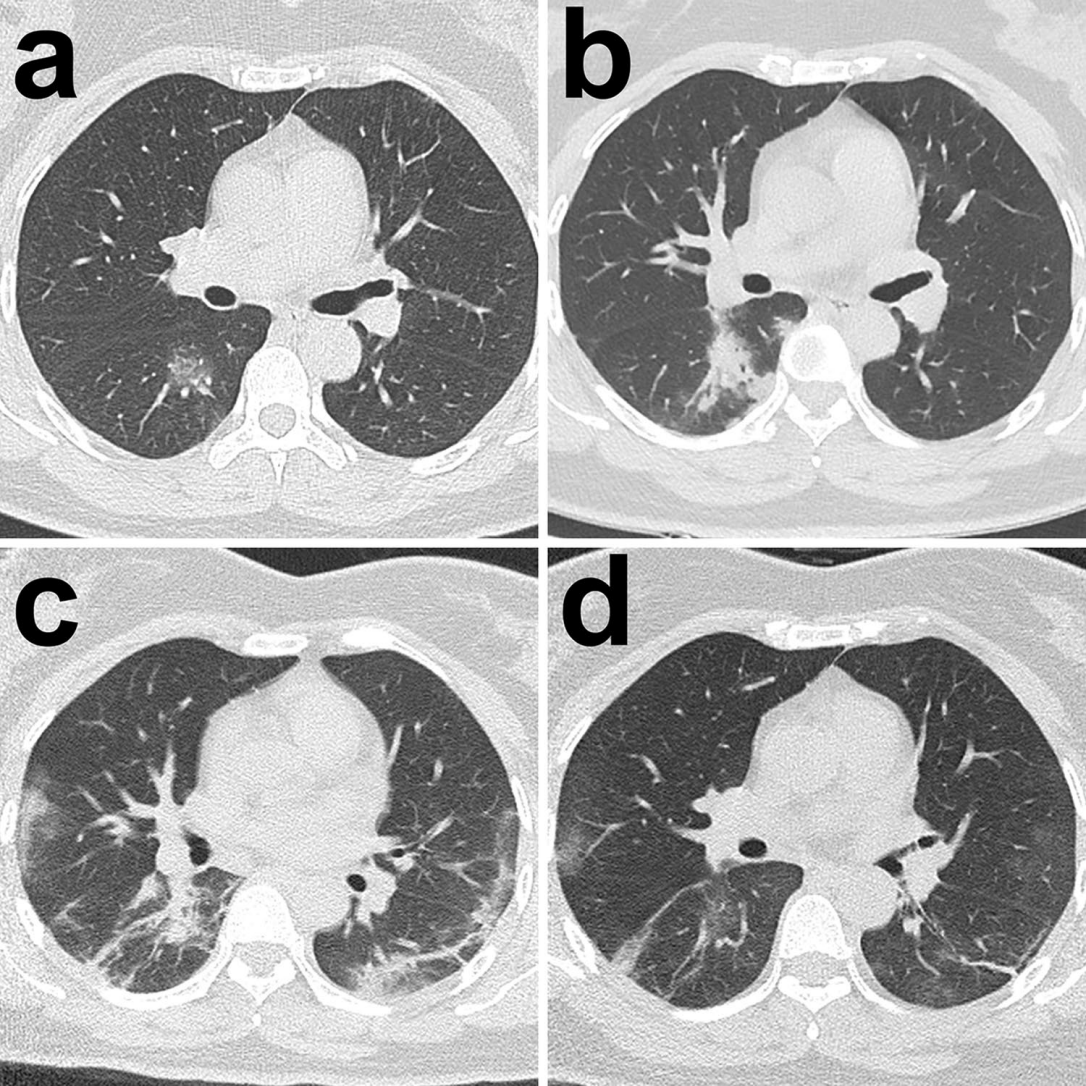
Typical radiological evolution of survivors. Images from a patient presenting with sudden fever (38.8 °C) for four days. (a) At presentation (day 4), a small region of GGO was demonstrated in the right lower lobe on CT scan and the RT-PCR test was performed afterwards; (b) on admission after confirming COVID-19 (day 9), the previous GGO became more consolidated with more surrounding subpleural lesions; (c) day 13, more bilateral subpleural GGO and consolidation were observed, and the previous consolidation was partially absorbed; (d) day 18, most of the lesions were absorbed while only some residual GGO and parenchymal bands could be observed. All images have the same window level of − 600 and window width of 1,600.
